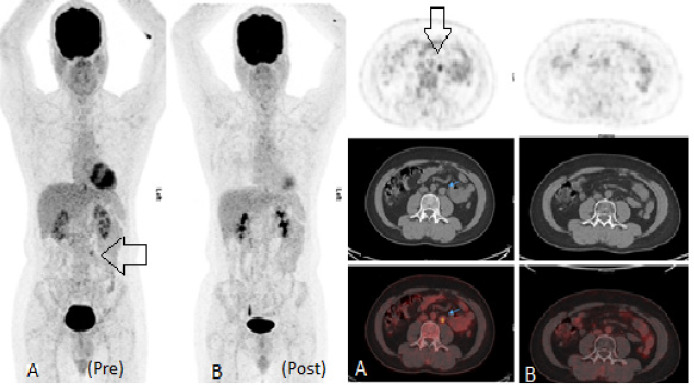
44-Years Man Underwent Orchidectomy for Seminoma of Left Testicle One Year Back. Follow up imaging showed solitary para-aortic lymph node which was difficult to characterize on CT scan as it was < 3cm, however FDG PET (A) confirmed its viability (arrows in Figure A) leading to start of treatment. (injected dose= 5.0 mCi) B) Post therapy PET CT showing complete resolution
